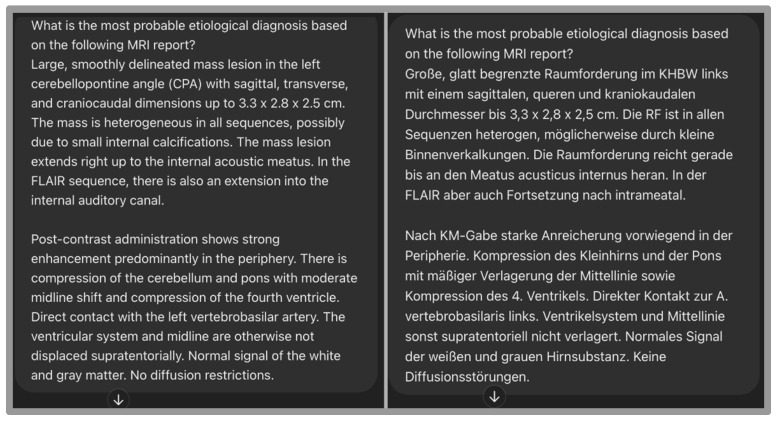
Example of the same prompt with MRI reports written in English and German (original language of the MRI report).
